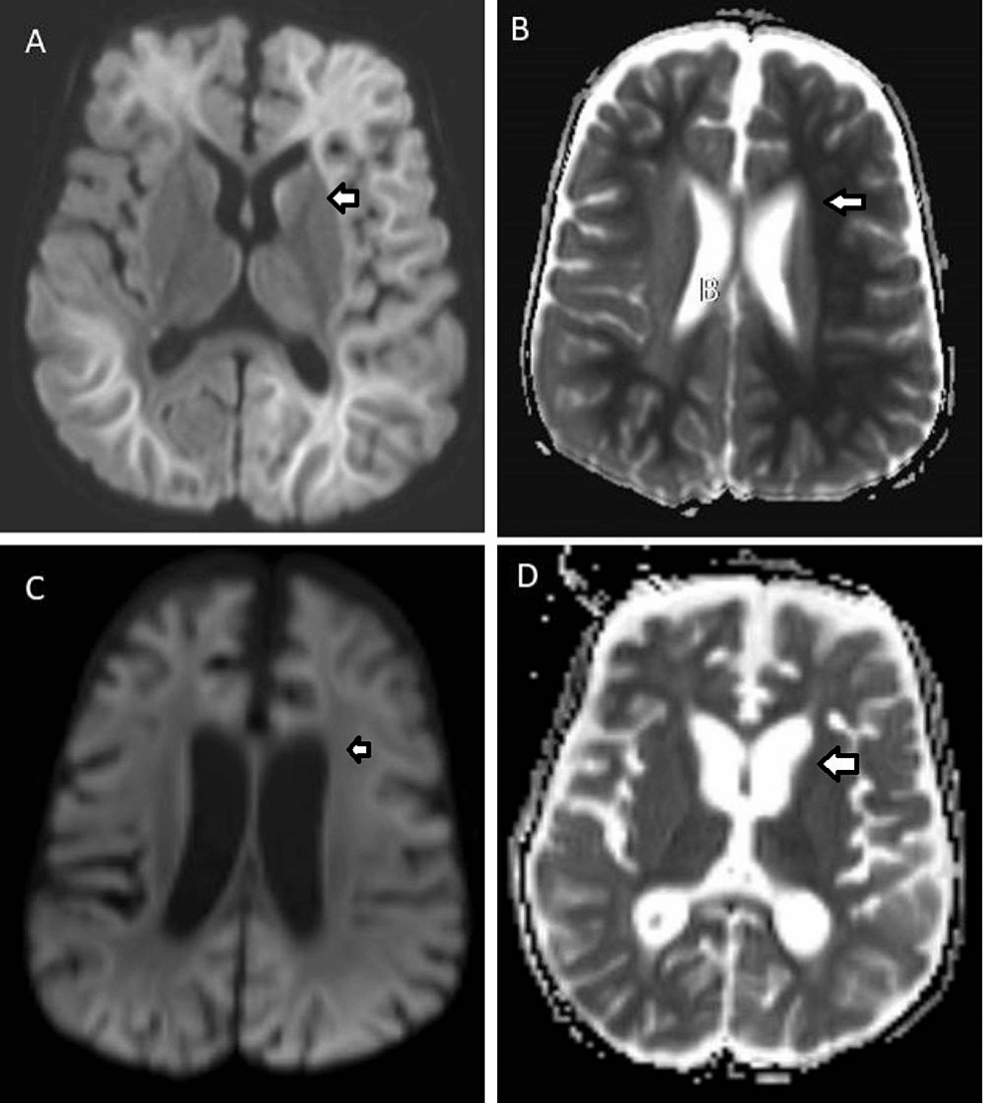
MRIs of the brain of the patient (arrows showing areas of interest) A. Diffusion-weighted MRI shows diffuse and confluent areas of diffusion restriction involving the bilateral cerebral white matter at the time of first presentation (arrow showing areas of interest) B. Apparent diffusion coefficient (ADC) map corresponding to the image in A shows a dark signal in the involved white matter, suggestive of true diffusion restriction (arrow showing areas of interest) C. Diffusion-weighted MRI two weeks after the initial presentation shows normalization of signal with no restricted diffusion in the white matter (arrow showing areas of interest) D. ADC map corresponding to C shows a normal signal in the white matter with no diffusion restriction (arrow showing areas of interest)
